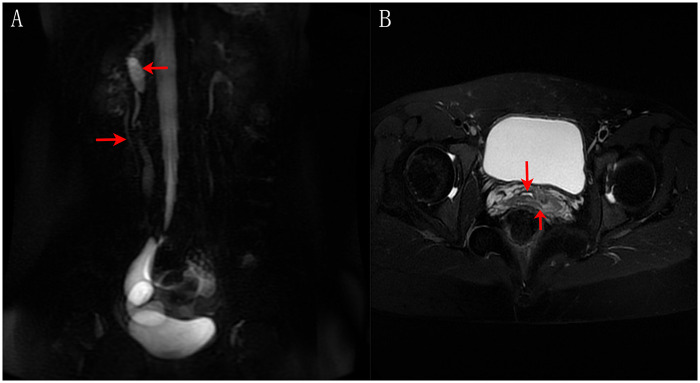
MRI of the urinary tract. (A) MR urography (MRU) shows a duplex kidney with ureteral duplication. The upper pole moiety and ureter are dilated (short arrows), and the lower pole moiety and ureter are normal (long arrows). (B) Axial T2-weighted fat-suppressed (T2WI-FS) image reveals tapering of the distal dilated ureter (long arrow) and its ectopic opening into the vagina (short arrow).
